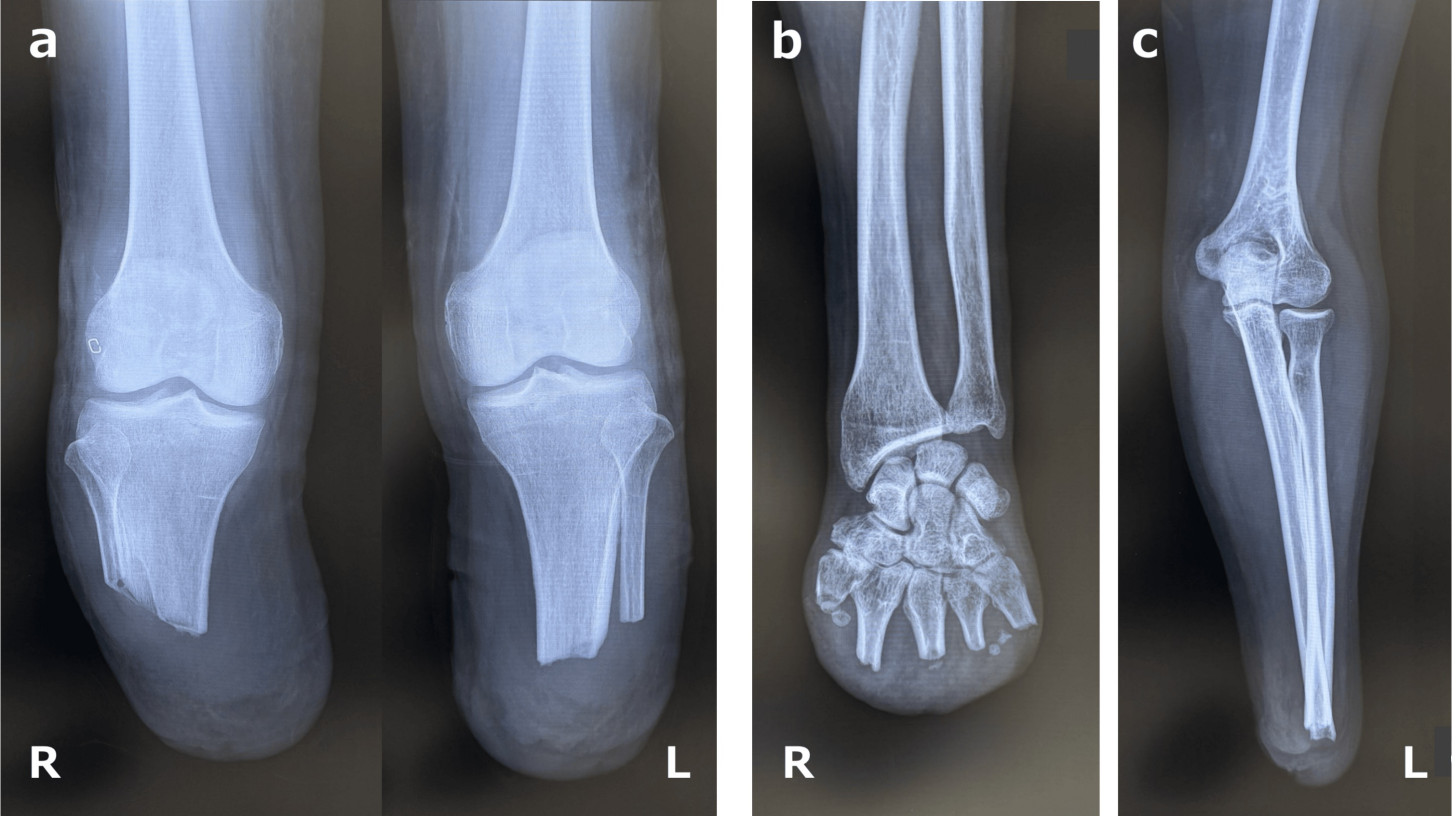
Plain radiographs of the stumps. (a) Bilateral below-knee amputation; (b) right partial hand amputation; (c) left below-elbow amputation.
